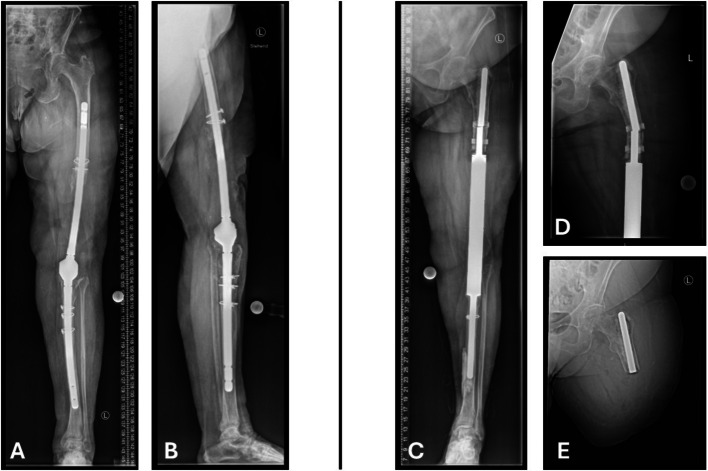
Representative radiographs showing an intact modular knee arthrodesis (MKA) (A, B) and a failed MKA due to a broken femoral stem (C, D), necessitating a consecutive proximal transfemoral amputation (E)
